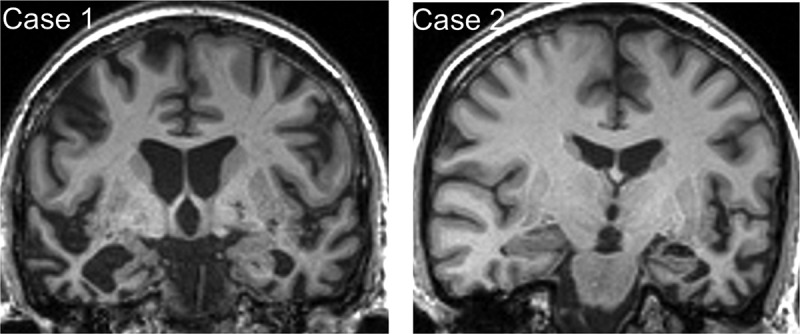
Brain MRI profiles in patients with semantic dementia and food aversion. Representative coronal T1-weighted MR sections through the anterior temporal lobes are presented for each of the patients described; the left hemisphere is shown on the right for both sections. In each case, there is relatively focal, asymmetric atrophy of the anterior temporal lobes, most marked medially and inferiorly (predominantly right-sided though bilateral in Case 1, predominantly left-sided in Case 2).
